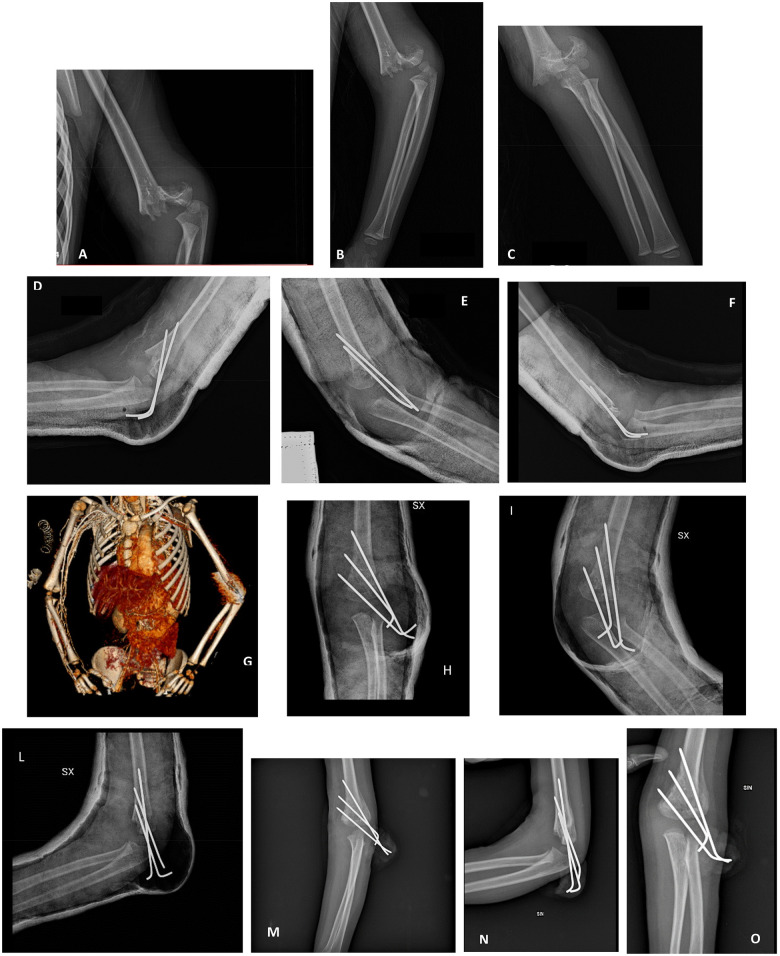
(A–C) 5-year-old male. Initial x-rays showed a Gartland type III supracondylar humeral fracture. On physical examination, deformity of the anatomical profile and functional impairment were observed. The radial pulse was present at admission, and a positive Kirmission sign was noted. The patient was poorly cooperative during the initial clinical evaluation. Given the high-energy mechanism of injury, an abdominal ultrasound and surgical evaluation were performed in the Emergency Department prior to admission to the Orthopedic Department. Approximately 12 h after admission, the radial pulse was no longer palpable on digital examination. Clinical assessment revealed anterior interosseous nerve deficit, characterized by inability to flex the interphalangeal joint of the thumb and the distal interphalangeal joint of the index finger, associated with hypoesthesia of the index finger. (D—F) Postoperative radiographs demonstrated two lateral, divergently placed Kirschner wires with bicortical fixation, providing stable fixation of both columns without convergence or crossing at the fracture site. The limb was then immobilized with a dorsal brachiometacarpal splint. (G) Contrast-enhanced CT angiography showed demonstrated occlusion of the distal brachial artery. (H—L) A surgical revision procedure was performed one week after initial presentation to optimize fracture fixation. Radiographic evaluation confirmed improved alignment and stabilization with three laterally inserted Kirschner wires. (M—O) At 1-month follow-up, callus formation and physiological remodeling were observed. Mild joint stiffness was noted. K-wires and cast were removed, and progressive mobilization was initiated.
